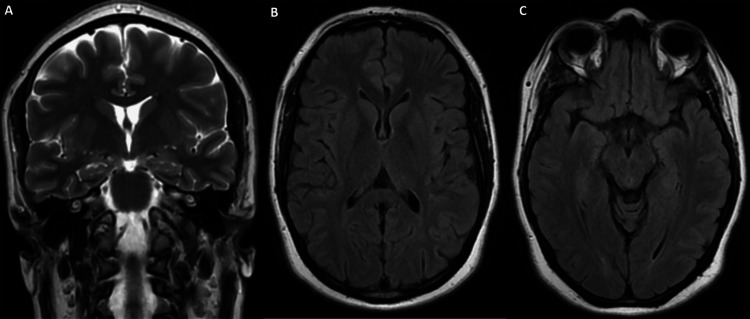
A: Coronal T2-weighted brain MRI showing disappearance of the lesion in the basal ganglia. B and C: Axial FLAIR brain MRI showing complete disappearance of lesions in the mammillary bodies and periaqueductal gray matter. MRI: magnetic resonance imaging, FLAIR: fluid-attenuated inversion recovery
